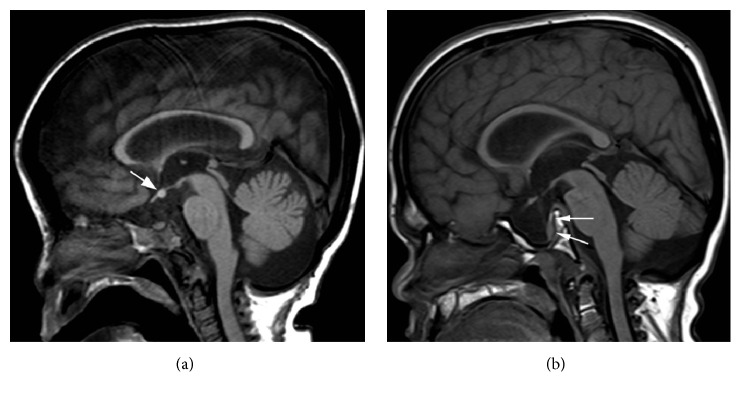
(a) Cranial MRI of Case 1 obtained at the age of 8 years reveals ectopic posterior pituitary lobe. Sagittal spin-echo T1-weighted image shows an abnormal, shallow sella turcica, as well as hypoplastic clivus. A normal T1-hyperintense posterior lobe of the pituitary gland is not identified in the sella turcica. Instead, a T1-hyperintense round structure (arrow) is noted inferior to the floor of third ventricle at the level of infundibular recess. A pituitary stalk is not seen. Prominent forehead related to macrocephaly is also noted. (b) Cranial MRI of Case 2 obtained at the age of 12 years reveals a large empty sella. Sagittal spin-echo T1-weighted image shows a markedly enlarged sella turcica filled with CSF. The anterior and posterior lobes of the pituitary gland (arrows) are displaced posteriorly. A hypoplastic clivus and prominent forehead are present.
